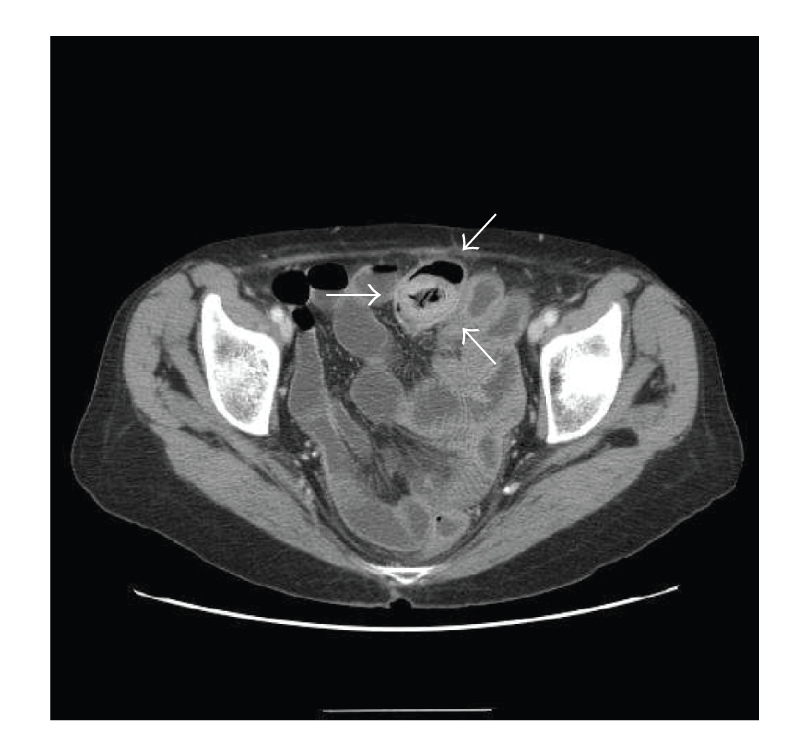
Axial abdominal CT scan showed an ovoid, nonhomogeneous, intraluminal filling defect about 3 cm in size (arrows). CT demonstrates no dilated small bowel loops, and the intraluminal mass with air retained in its interstices, which correspond to the phytobezoar.
